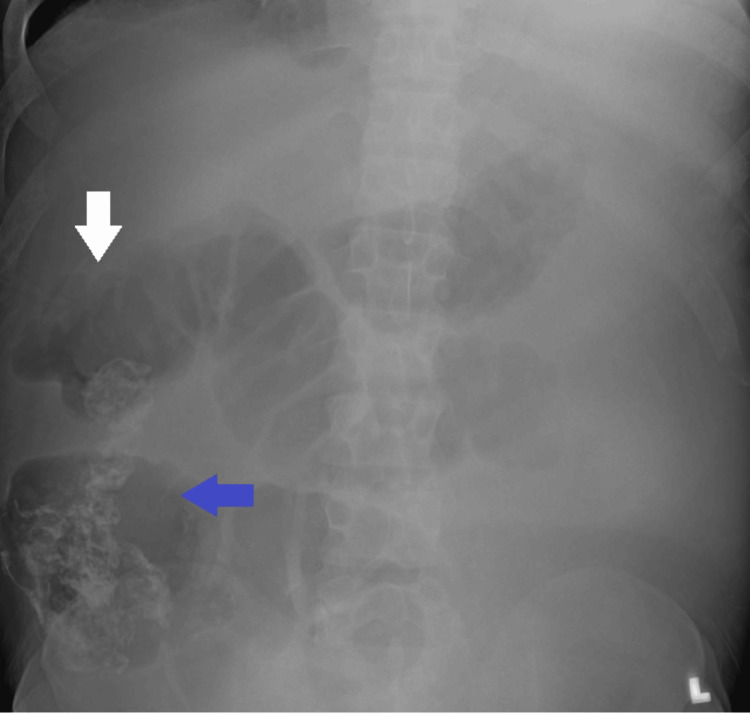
X-ray abdomen showed distention of the cecum (blue arrow) and distension of ascending colon (white arrow)
